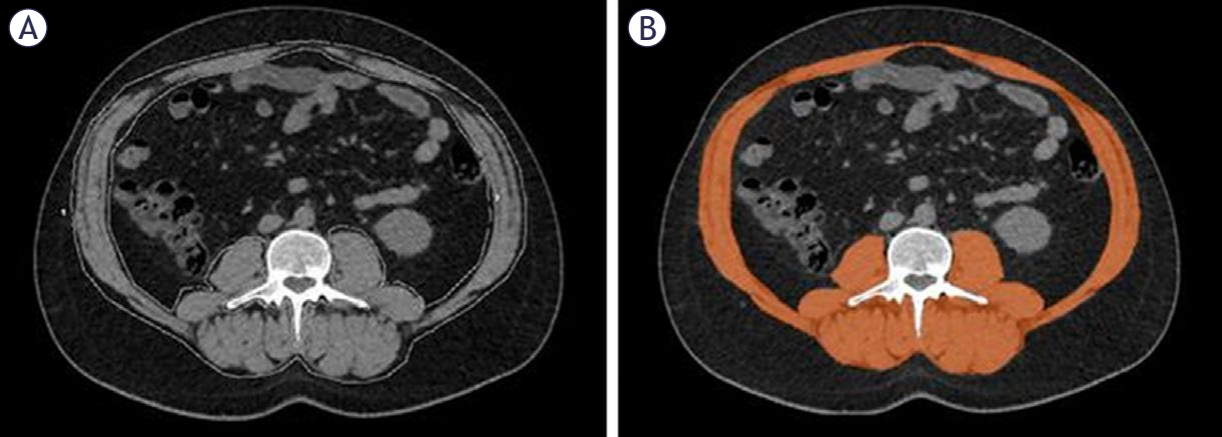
Axial computed tomography images at the level of the third lumbar vertebra. (A) Unprocessed image used for skeletal muscle area analysis. (B) Skeletal muscle areas (highlighted in orange) were manually segmented based on a Hounsfield unit (HU) threshold range of -29 to +150 to quantify muscle tissue area.
